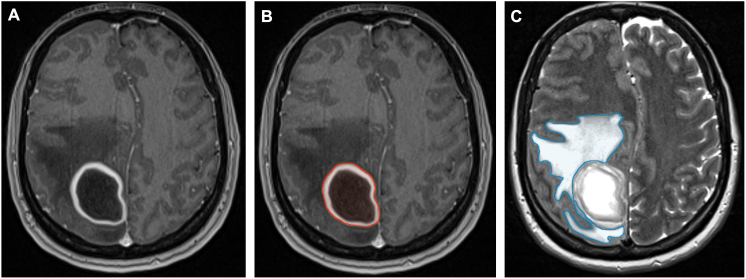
Representative cranial MRI sequences of a brain abscess. A: Axial T1-weighted contrast-enhanced sequence demonstrating a right parietal brain abscess. B: Segmentation of abscess volume (outlined in red) on contrast-enhanced T1-weighted MRI. C: Segmentation of perilesional edema volume (outlined in blue) on T2-weighted MRI.
